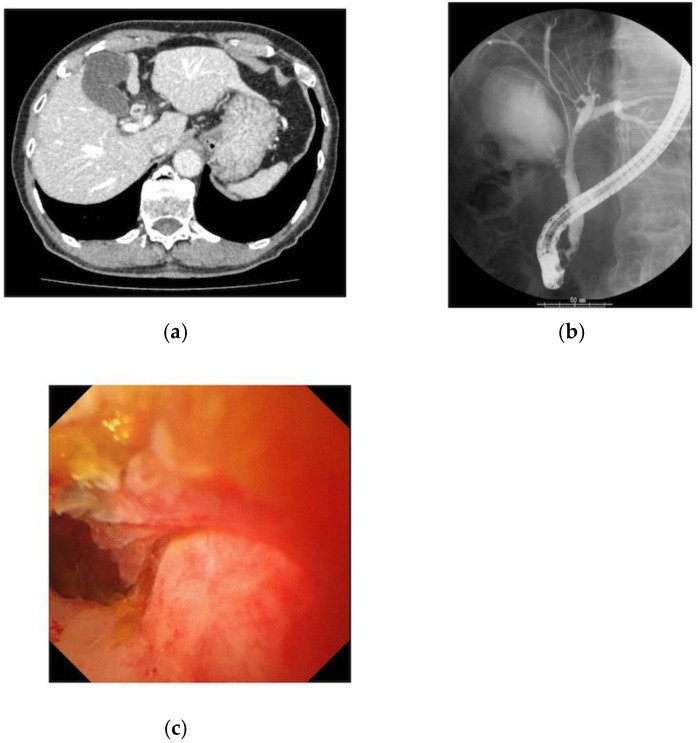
Images of the case with bile wall thickness which was finally diagnosed as IgG4SC: (a) The CT scan revealed wall thickening in the common bile duct, with nodular thickening of the bile ducts in the hilar region; (b) the ERCP showed that the wall of the bile duct was hard, and the mucosal edge was rough from the cystic duct to the hilar region of the bile duct; (c) cholangioscopy showed rough mucosa with irregular papillary elevation.
